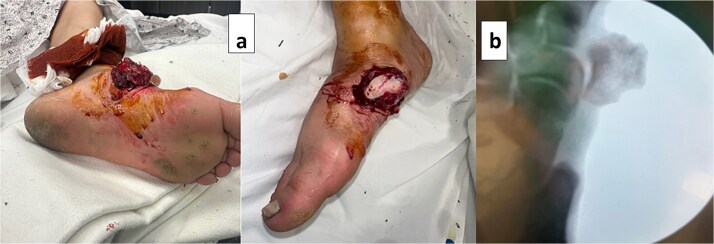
(a) Clinical photograph demonstrating an open dorsomedial wound of the right midfoot with exposed bone and articular cartilage. (b) Initial radiograph demonstrating dorsomedial displacement of the medial cuneiform relative to the navicular and base of the first metatarsal, widening of the first tarsometatarsal interval, and disruption of the medial-intermediate intercuneiform articulation.
